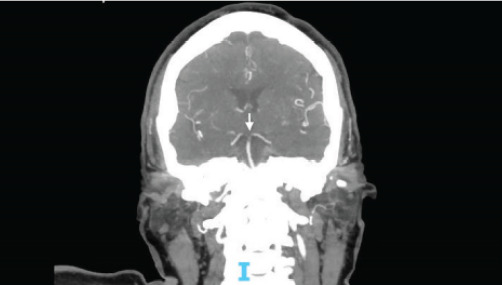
Computed tomography angiography coronal view (Patient 2) with arrow pointing to the site of basilar artery occlusion with bilateral posterior cerebral artery involvement.
